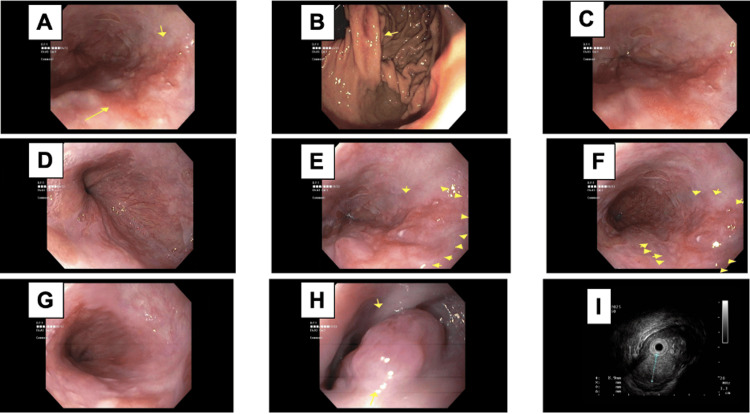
Serial endoscopic evaluations of the distal esophagus across three EGDs In all panels, yellow arrows identify specific mucosal features of interest, and yellow arrowheads delineate the borders of abnormal mucosa. (A-C) EGD1 (February 2025): (A) lower third of the esophagus, with yellow arrows highlighting salmon-colored columnar mucosa consistent with Barrett's esophagus; (B) yellow arrow indicating endoscopic evidence of the prior Nissen fundoplication wrap at the gastroesophageal junction; (C) gastroesophageal junction with subtle mucosal nodularity and irregularity extending from 37-38 cm from the incisors, subsequently shown to harbor invasive squamous cell carcinoma on biopsy. (D-F) EGD2 (March 2025): (D) gastric cardia with Barrett's mucosa before resection; (E–F) abnormal mucosa in the lower third of the esophagus viewed from two different angles, with yellow arrowheads delineating the lesional margins targeted for endoscopic mucosal resection, which was subsequently performed en bloc. The squamous cell carcinoma in situ lesion was located at 37–38 cm from the incisors and was non-circumferential. (G-I) EGD3 (July 2025, surveillance): (G) well-healed post-EMR scar at the Z-line; (H) new friable nodular mass at 34 cm from the incisors, with yellow arrows identifying the lesion; (I) endoscopic ultrasound of the distal esophageal lesion EGDs: esophagogastroduodenoscopies; EMR: endoscopic mucosal resection
